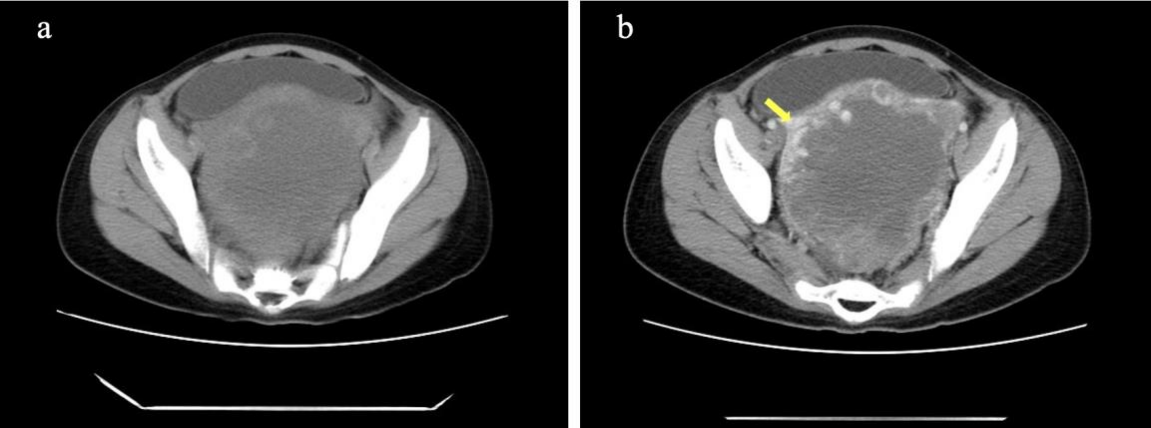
Non-contrast and contrast-enhanced CT image description. Axial non-contrast (a) and contrast-enhanced (b) CT images at the abdominal level demonstrating a heterogeneously enhancing mass with pronounced enhancement of the peripheral solid component (yellow arrow) and absence of enhancement in the central necrotic area.
